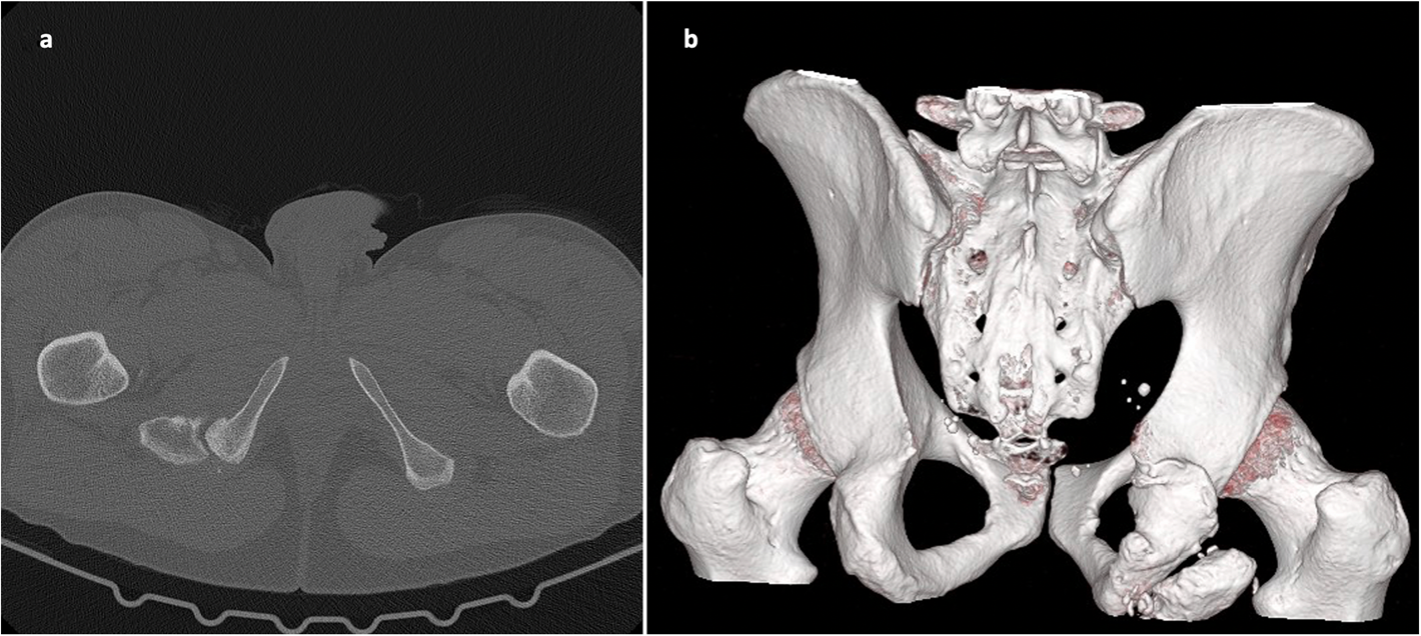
Preoperative CT showed nonunion of the displaced fragment (a) and location of the fragment in preoperative three-dimensional CT (b)
